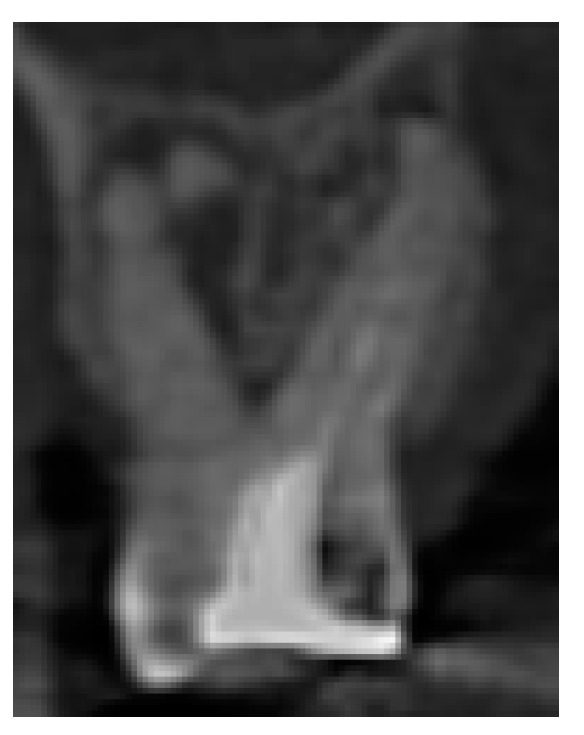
P—the teeth with periapical lesion.
